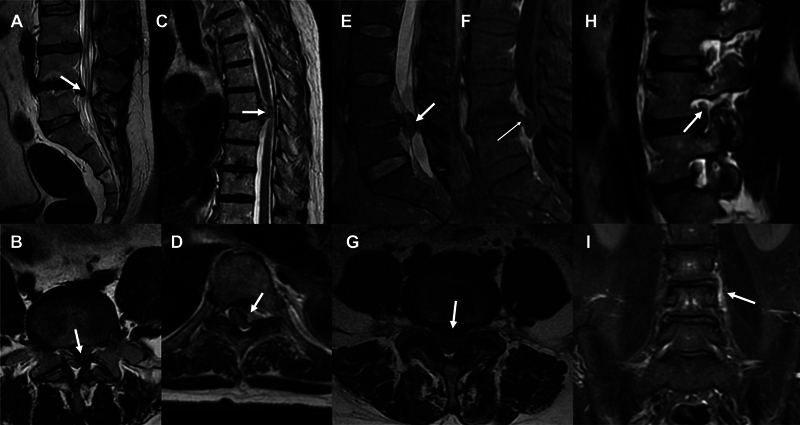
Variety of intervertebral disc hernias detected by MRI in patients with neuropathic pain. Sagittal (
